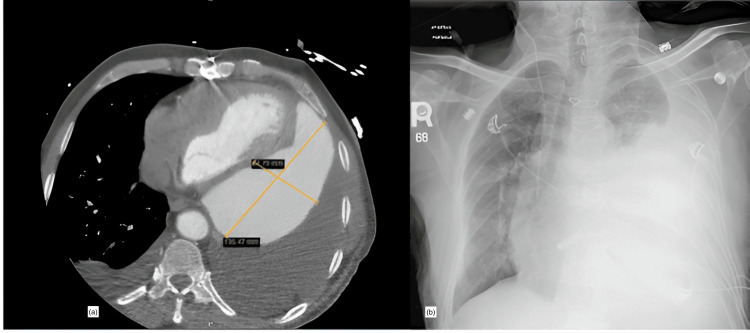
Axial views of the chest computed tomography scan reveal a contained left ventricle rupture and hemopericardium (a), and the PA view of the chest X-ray (b) reveal near total left lung compression secondary to a large serosanguineous left pleural effusion
